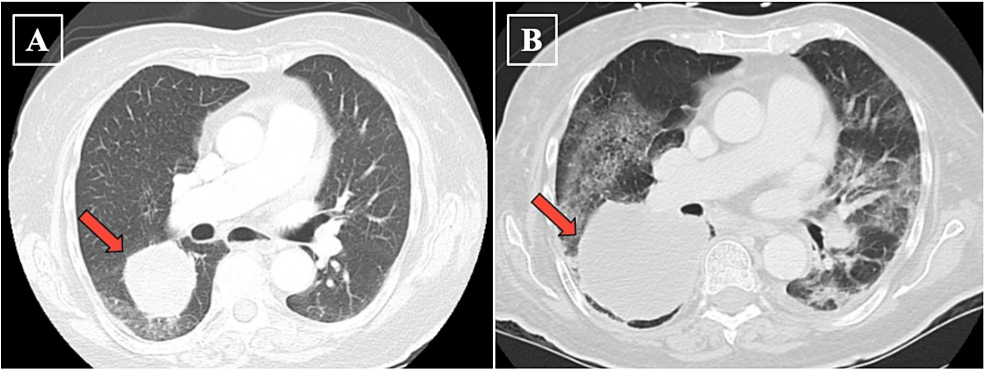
A: CT chest from 132 days prior to hospital admission CT chest (seen in pane B), demonstrating a right lower lobe lung mass (red arrow). B: CT chest obtained on day 9 of hospital admission demonstrating a larger right lower lobe lung mass (red arrow).
